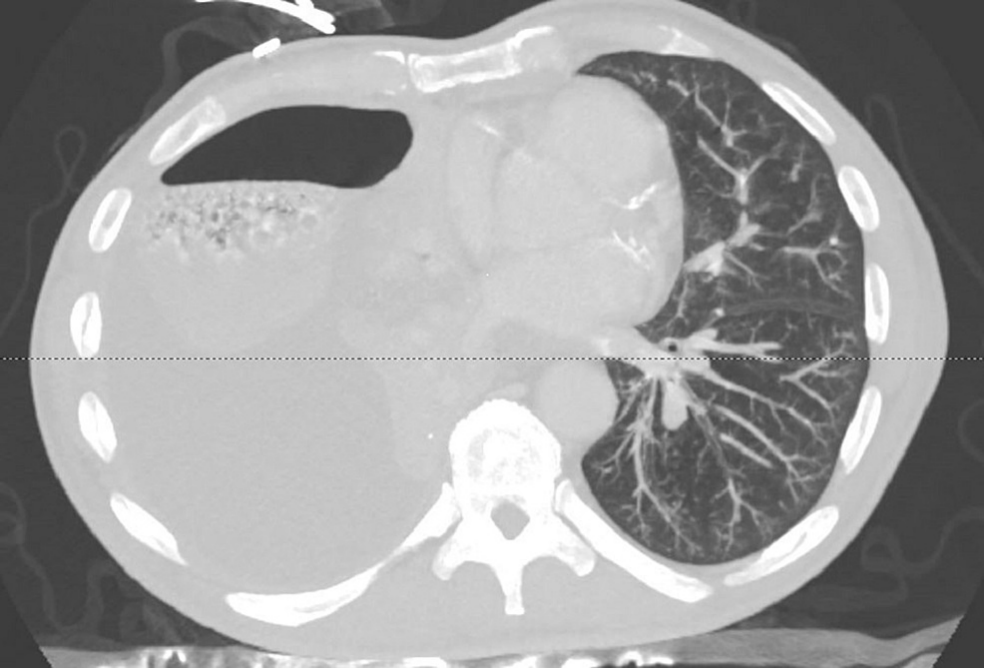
Transverse view of CT chest showing a large right hydropneumothorax with a near-complete filling of the right hemithorax
